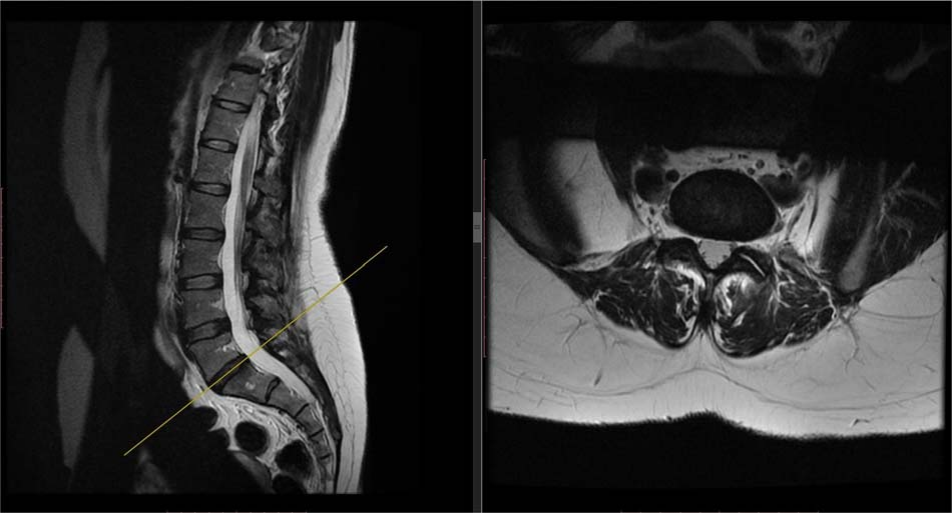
Preoperative MRI of the lumbar spine performed in December 2016. The MRI shows no neurological compression.
